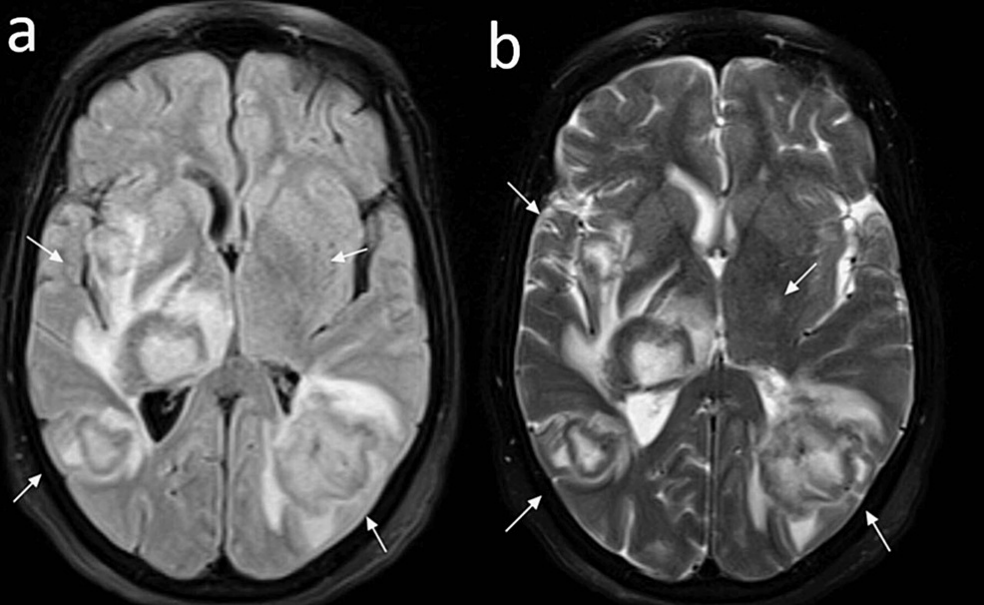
Brain magnetic resonance imaging Axial susceptibility-weighted imaging images showed multifocal non-confluent white matter lesions seen in both cerebral hemispheres having a central bright fluid-attenuated inversion recovery (FLAIR) signal intensity and peripheral dark FLAIR signal (a). Hemorrhagic components and restriction are seen as well on diffusion-weighted imaging (DWI) at the periphery of the lesions (b). Marked perilesional edema is noted. Involvement of the right thalamus with surrounding edema and some compression of the adjacent midbrain and the third ventricle causing mild entrapment of the frontal horn of the right lateral ventricle. This pattern of multifocal lesions with surrounding edema has been described in COVID post viral infection brain hemorrhagic lesions. Appearance can be like hemorrhagic acute disseminated encephalomyelitis.
